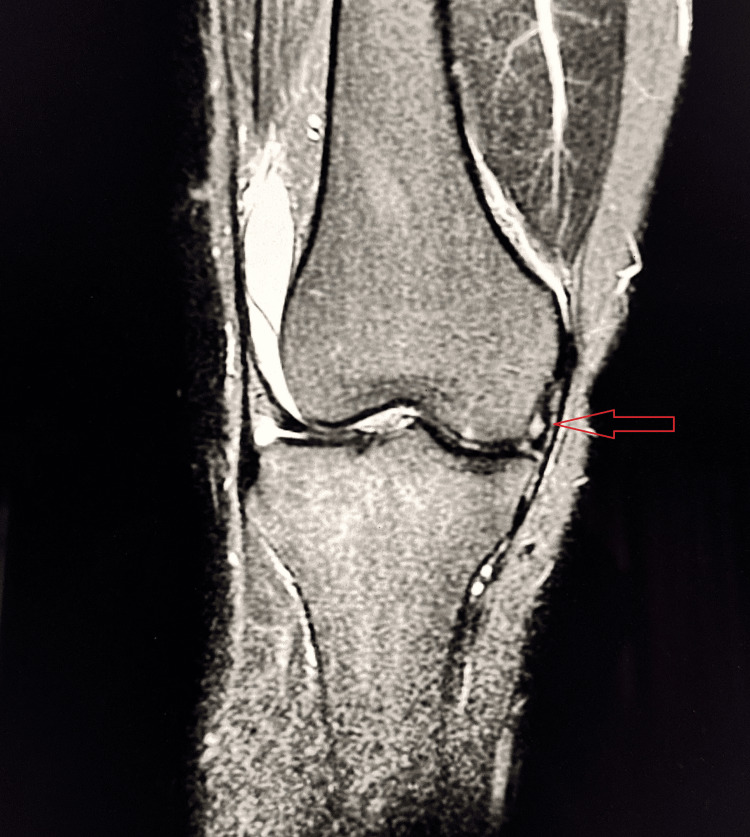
Grade I injury of the MCL Coronal T2-weighted MRI demonstrates thickening and hyperintensity of the MCL without fiber discontinuity, consistent with Grade I MCL sprain (red arrow). Mild joint effusion is present. MCL, medial collateral ligament
